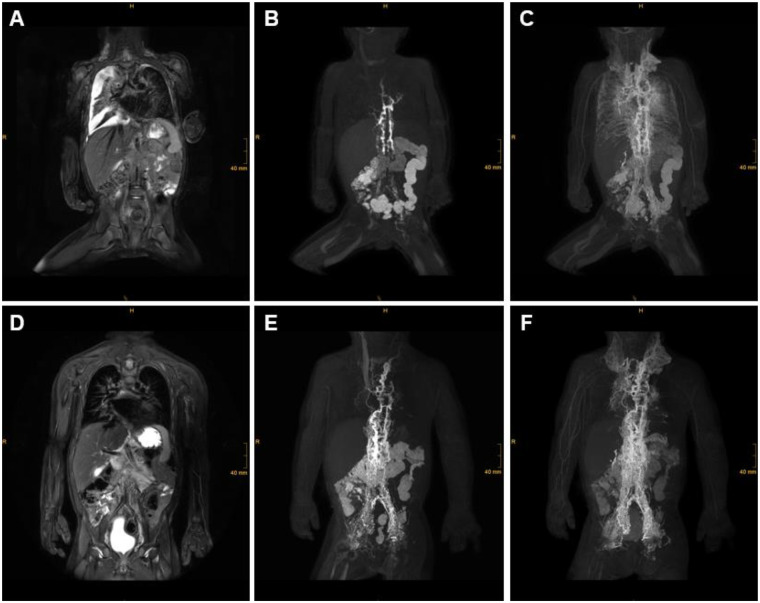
MR lymphangiography of a 2-year-old boy with noonan syndrome (*RIT1*-pathogenic variant) and refractory chylothorax. Coronal T2-weighted mDixon image (water image) shows a large pleural effusion on the right (A) Coronal maximum intensity projections (MIP) of a contrast-enhanced MR-lymphangiogram in the early phase shows tortuous lymphatic vessels without a continuous thoracic duct (B) This results in massive reflux into the pleural, pulmonary, and cervical lymph vessels in the late phase (C) After one year of treatment with Trametinib, T2-weighted imaging shows resolution of chylothorax (D) The early phase of contrast enhancement (E) continues to show evidence of the markedly tortuous and progressively dilated vessel in the position of the thoracic duct. The late phase shows substantially reduced, but still visible chylo-lymphatic reflux into mediastinal and cervical lymph vessels (F) Especially reflux into intercostal/pleural lymphatics is considerably reduced after one year of treatment.
